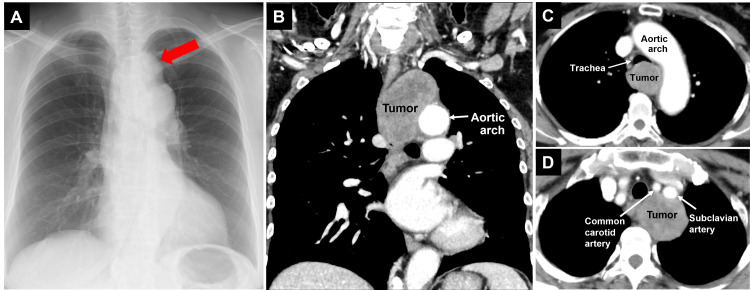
Chest radiograph and computed tomography image before surgery Chest radiograph showing a mass shadow above the first left arch (red arrow in A). A 4.6×4.3×6.5 cm posterior mediastinal tumor was compressing the trachea and left main bronchus, causing approximately 50% stenosis at the tracheal carina (B, C). The tumor was in contact with the esophagus, thoracic vertebrae, aortic arch, left subclavian artery, and azygos venous arch, though the invasion into them was not obvious (B-D).
